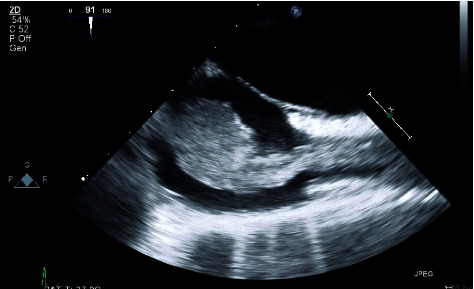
Transesophageal Echocardiogram (TEE) midesophageal bicaval view: large lobulated mobile mass extending from the superior vena cava (SVC) into the right atrium.
